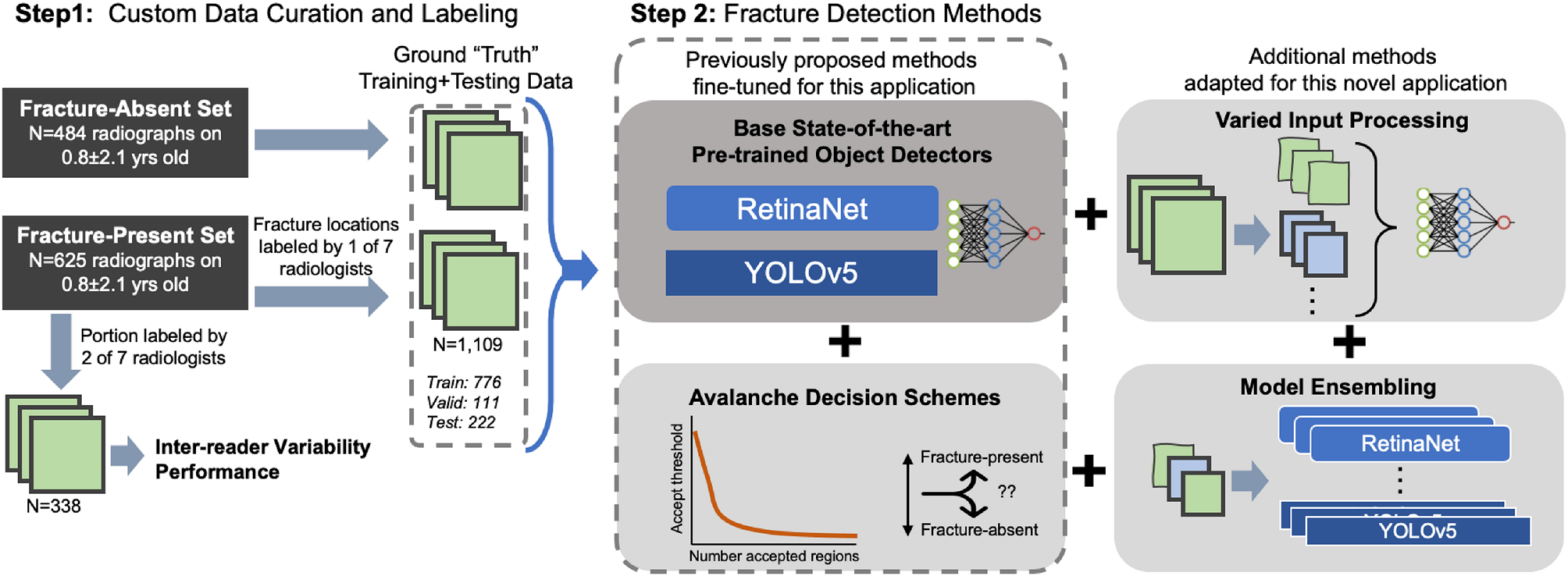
Summary of the major contributions of this paper including curation of a labeled dataset and the addition of up to three high-sensitivity methods (avalanche decision scheme, varied-input processing, and ensembling) to start-of-the-art pre-trained object detectors. In addition, inter-reader variability between expert radiologists was evaluated on 338 of the 624 fracture-present radiographs.
